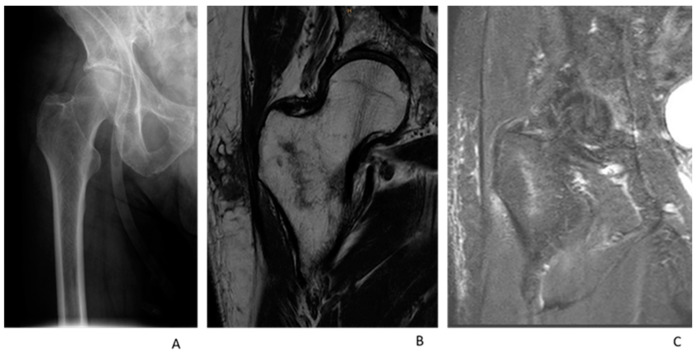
An 85-year-old man with severe hip pain after a ground-level fall. (A): AP Radiograph showing no fracture; (B): T1 Coronar Sequence MRI showing intertrochanteric fracture line; (C): STIR Coronar Sequence showing intertrochanteric oedema.
